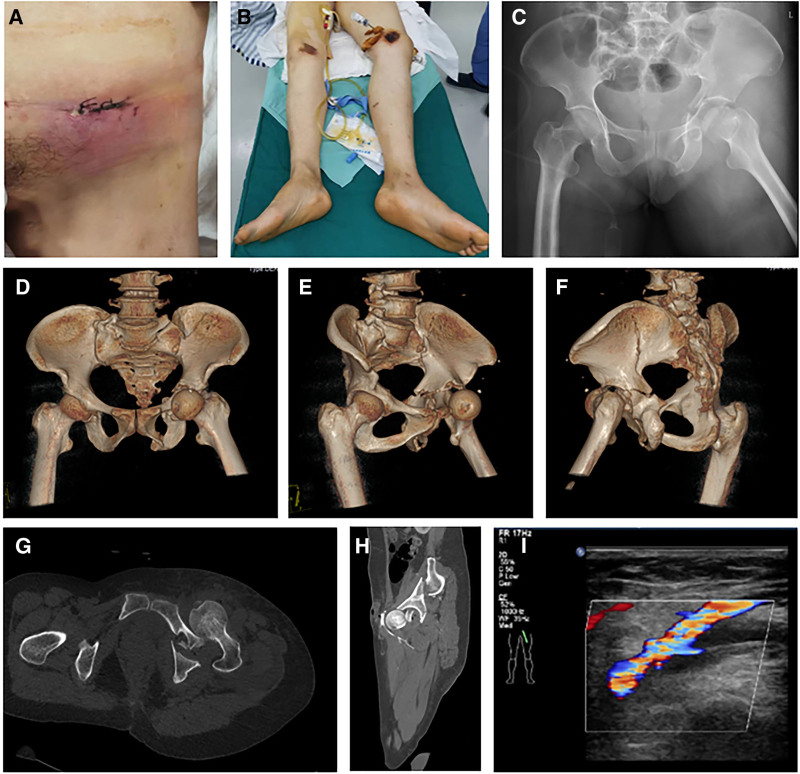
(A) The appearance of the patient’s left inguinal area wound on admission. (B) The patient’s left lower limb was in external rotation deformity before reduction. (C–F) The X-ray and computed tomography (CT) images on admission showed the femoral head in anterior dislocation and acetabular fracture (letournel-judet T shaped). (G) The CT tomography image on admission showed an avulsion fracture of the femoral head. (H) The CT angiography image on admission showed the dislocated femoral head compressing the common femoral artery. (I) The Doppler ultrasound image of the lower limb veins on admission showed a filling defect of the blood flow signal in the common femoral vein, indicating thrombosis.
